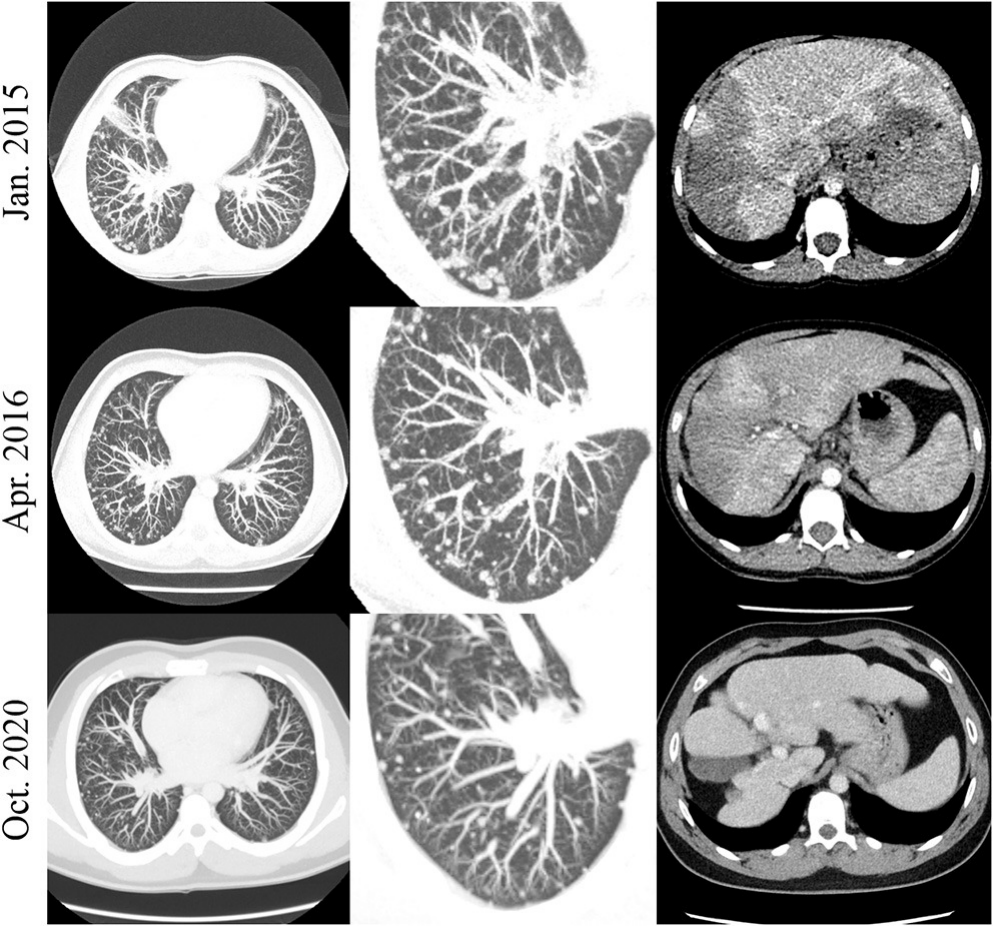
Patient #16. Exemplary chest (left and middle row) and abdomen (right row) CT slices in patient #16. A decrease in the amount and size of the numerous nodular lung lesions is noted over time. The initially hypodense liver lesions were involuted over the course of the examinations.
